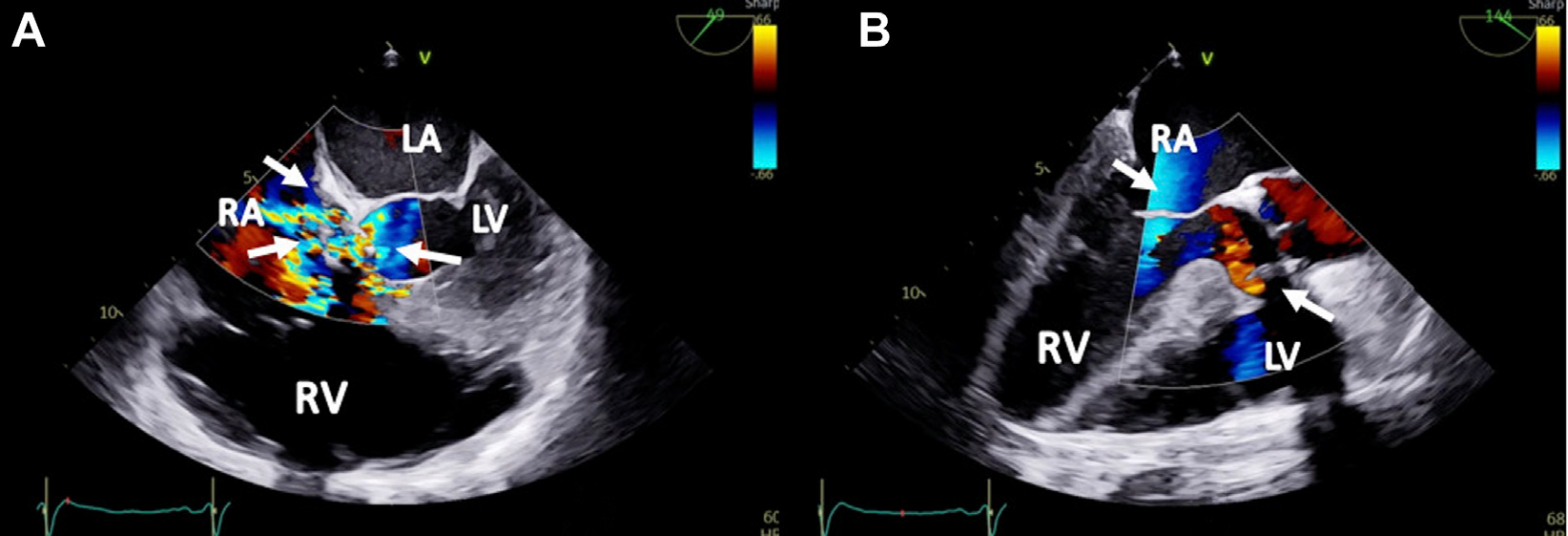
Transesophageal Echocardiographic images (A) Midesophageal 4-chamber view showing a Gerbode defect with color flow between the left ventricle (LV) and the right atrium (RA), with systolic color flow. (B) Long-axis view with color flow Doppler showing abnormal flow between the left ventricle and the right atrium. Abbreviations as in Figures 2 and 3.
